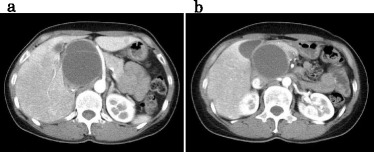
a, b Computed tomography images showing a recurrent cystic mass with septa in the same region of the first operation
